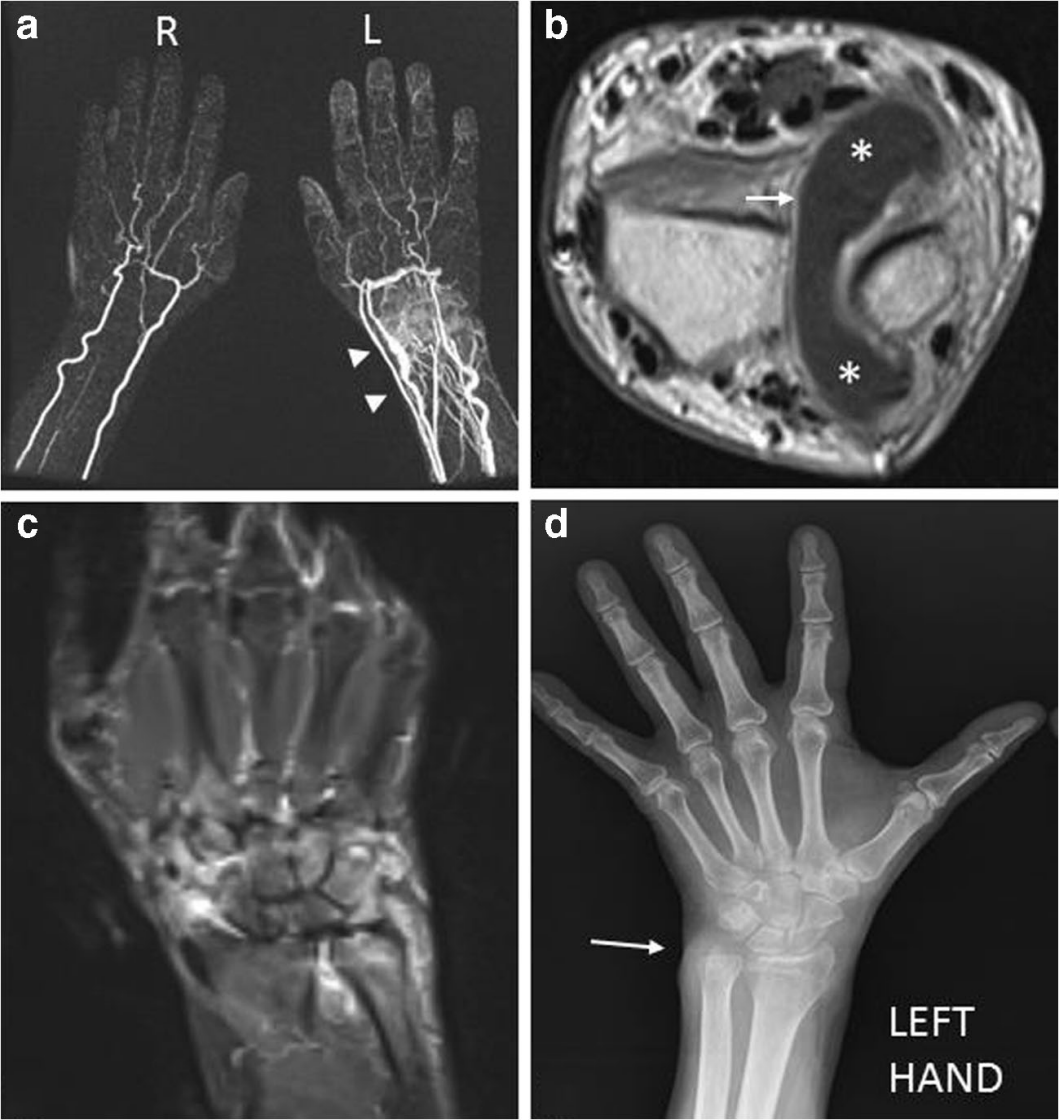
a, b, c, d Inflammatory. A 56-year-old rheumatoid arthritis (RA) male patient with pain and swelling in the left wrist. TWIST Angio 3D MIP imaging (a) clearly shows the inflammation with hypervascularization and edema (*arrowheads*) affecting the left wrist. Contrast-enhanced T1w imaging (b) demonstrates an abundant intra-articular effusion in the distal radioulnar joint (*) with thickening and enhancement of synovia (*arrow*), a condition referable to inflammation in the active phase. STIR coronal imaging (c) highlights the ELMSI affecting part of the ulna, lunate, triquetrum, hamate and capitate bones, and the effusion in radioscaphoid and radioulnar joints. Finally, the X-rays of the hand (d) taken at the same time shows a “soft-tissue sign” near the ulnar styloid process (*arrow*). but absence of erosions, while MRI is strongly positive for active inflammation [courtesy of Giovanni D'Elia, MD, Careggi University Hospital, Florence, Italy]
